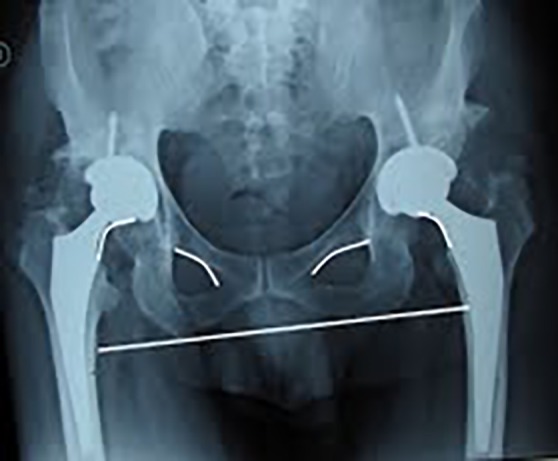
Post-op bilateral pelvic X-ray showing the equally spaced broken Shenton’s lines.
